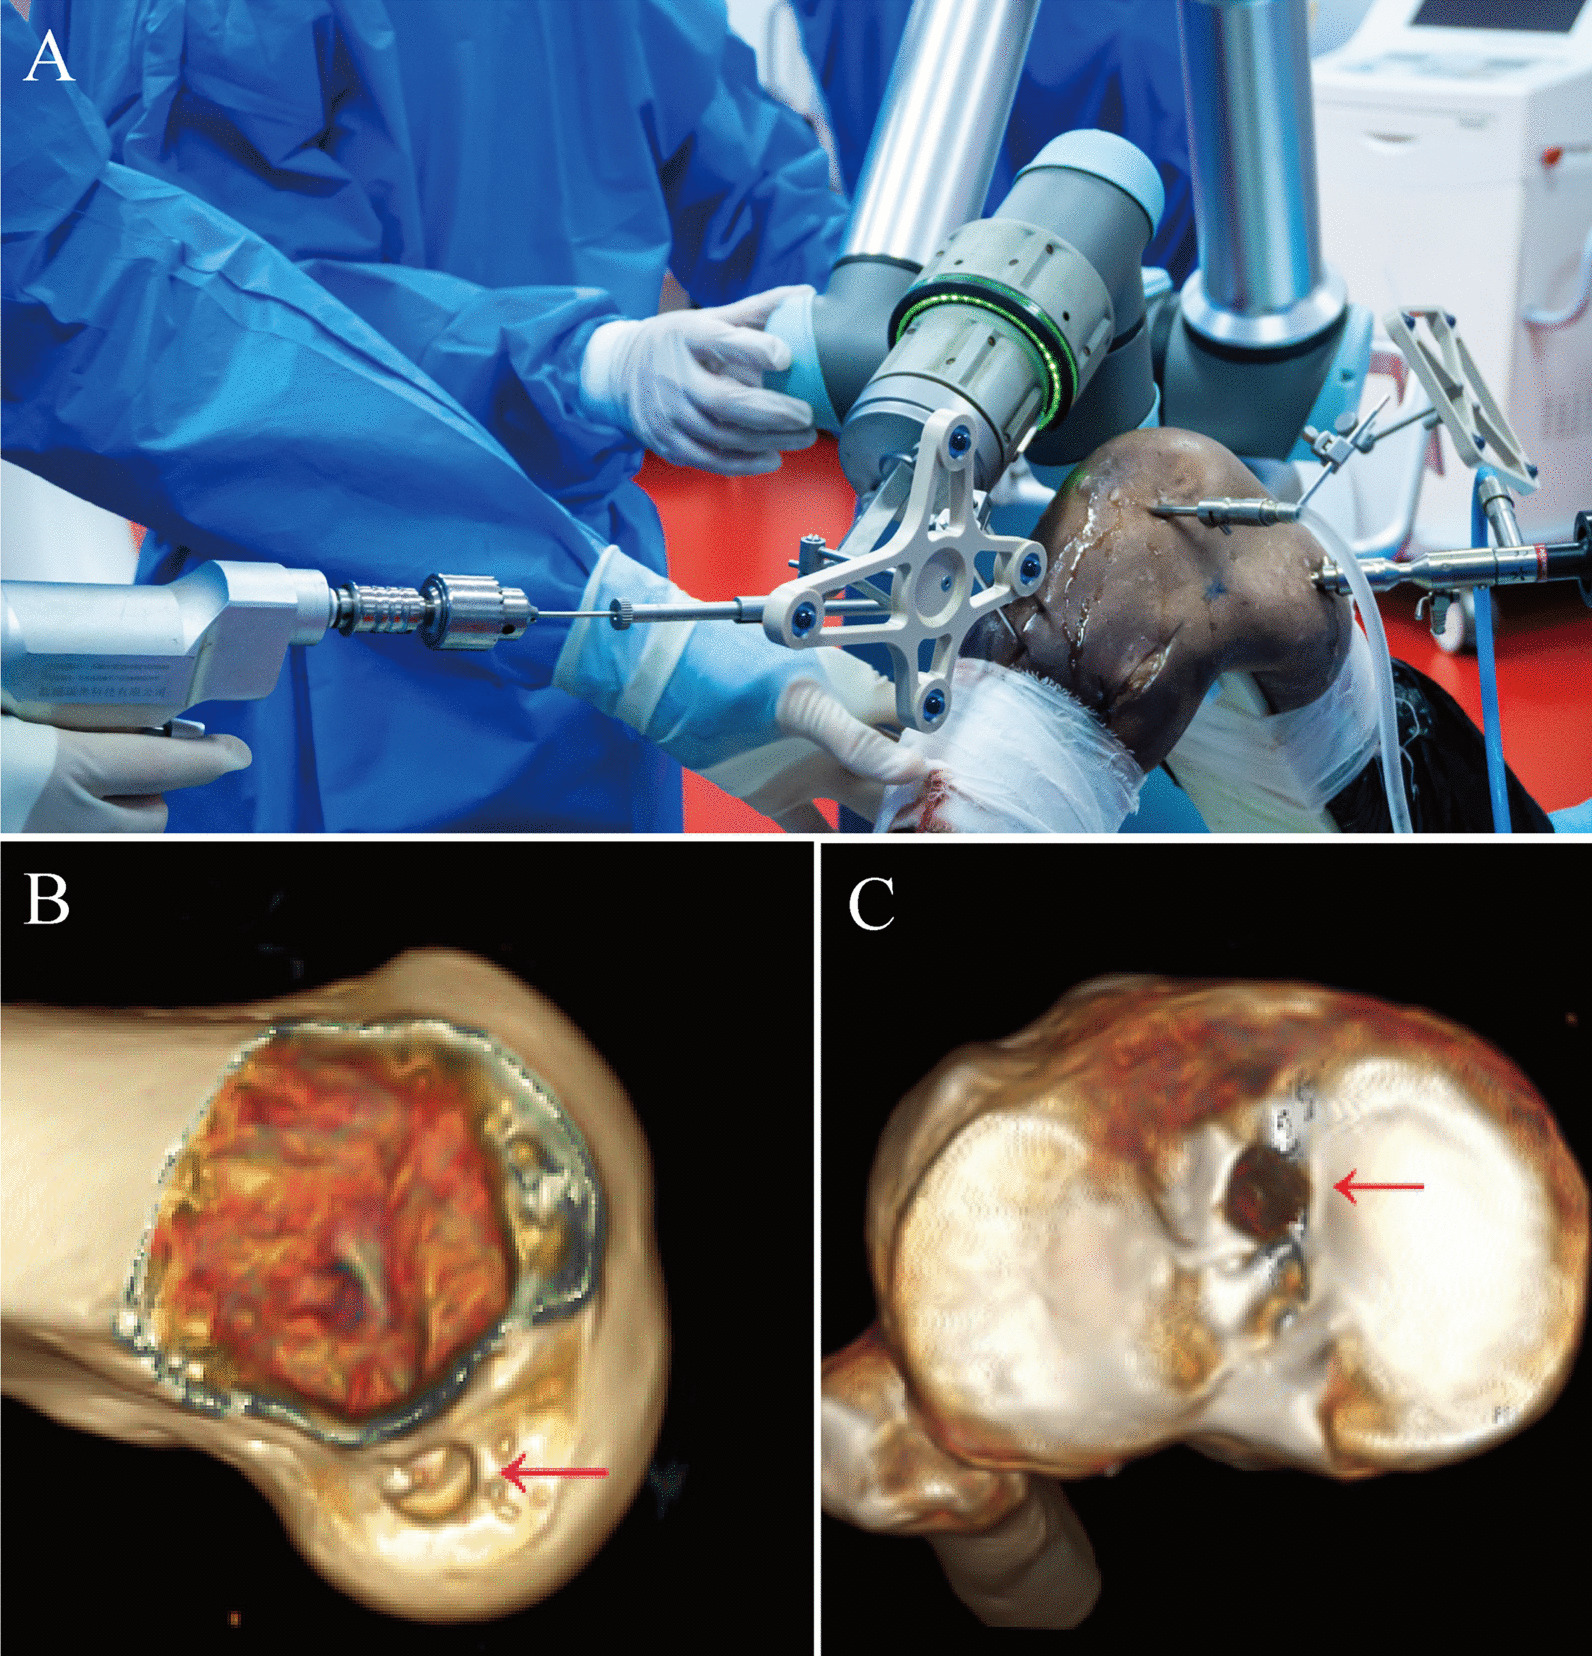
Tunnel location as shown in the arthroscopic field and 3D CT images. A After the robot arm completes the positioning step, the doctor drills the bone tunnel; B the location of the intra-articular opening of the femoral tunnel on 3D CT; C the location of the intra-articular opening of the tibial tunnel on 3D CT
